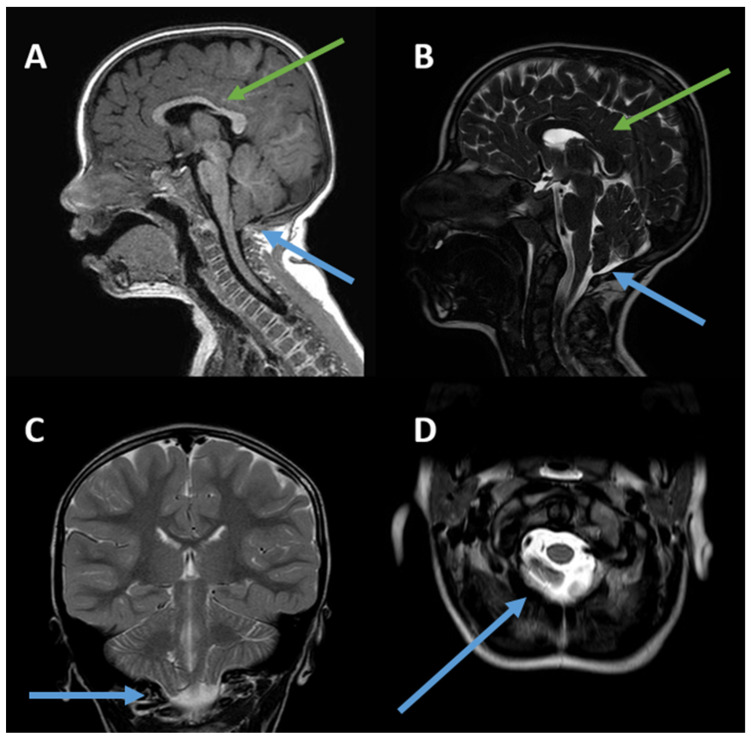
(A–D). Chiari I malformation and slightly thinned appearance of the body/splenion junction of the corpus callosum. (A) Sagittal 3D TFE T1-weighted image; (B) sagittal T2-weighted image; (C) coronal T2-weighted image; (D) axial T2-weighted image. The green arrows indicate thinning of the corpus callosum, while the blue arrows refer to Chiari I malformation. The posterior fossa is small. The peg-like cerebellar tonsils (C) herniate into the foramen magnum (D). Tonsillar ectopia is more pronounced on the right (C). The axial image shows the tonsils (D) behind the medulla oblongata, which cause crowding of the foramen magnum.
